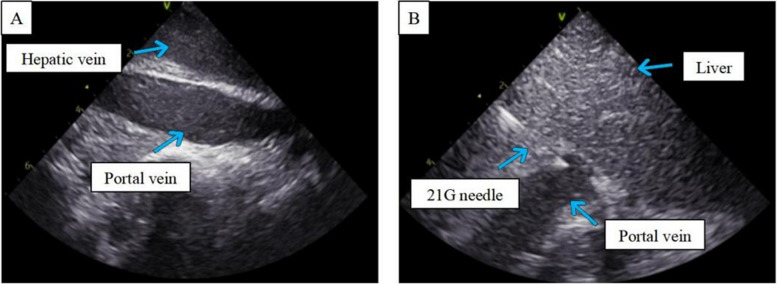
Ultrasound images captured in vivo with IVNU. A Right medial hepatic vein. B Right medial hepatic vein punctured by an IVNU-guided 21G needle
